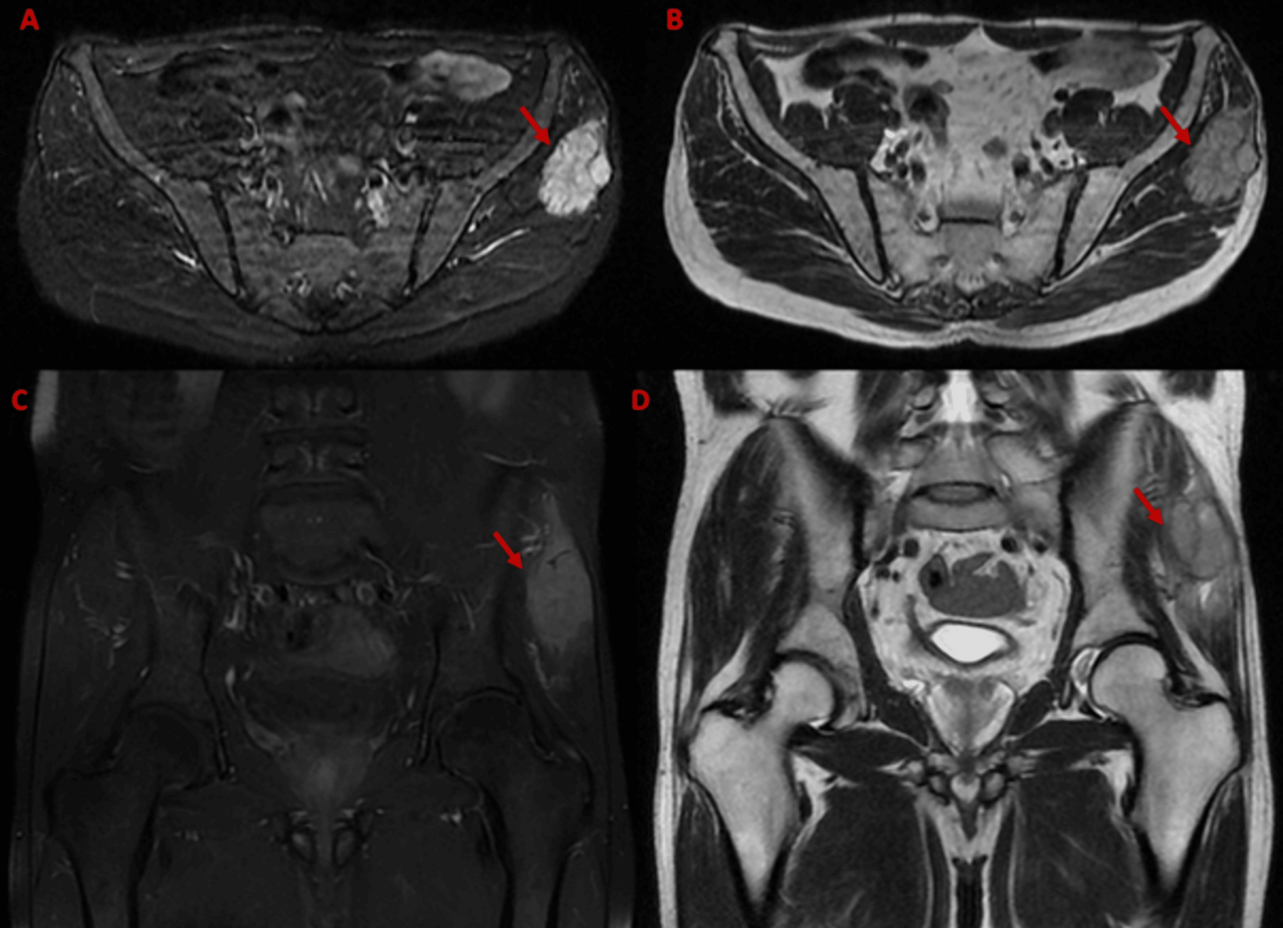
MRI evaluation of the right gluteal mass. (A) Axial T2-weighted fat-suppressed MRI sequence showing a hyperintense soft tissue lesion in the right gluteus maximus. (B) Axial T1-weighted image showing the lesion as isointense. (C) Coronal T2-weighted fat-suppressed sequence confirming the hyperintense signal of the lesion. (D) Coronal T1-weighted image revealing an isointense mass in the same location.
